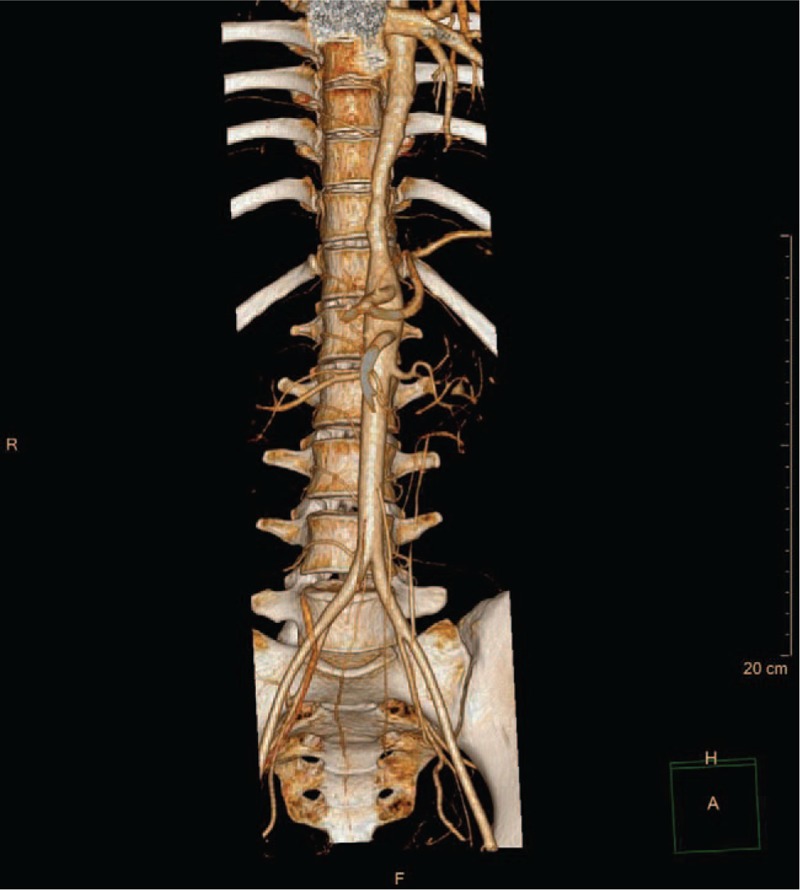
CTA of thoracoabdominal aorta. CTA showed stenosis in the thoracoabdominal aorta.
